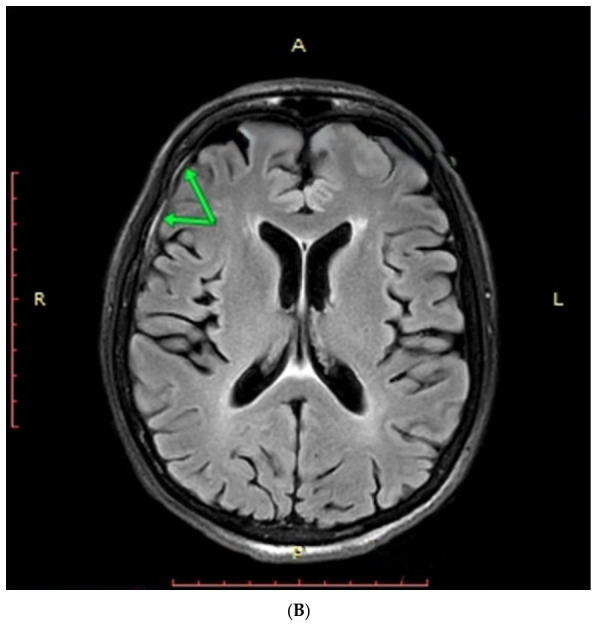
(A) Axial section of the brain on non-contrast computed tomography (radiological image of a right-sided subdural hematoma causing marked compression of the brain tissue—measurement of the hematoma width). (B) Axial section of the brain in T2 FLAIR sequence on 3T MRI (radiological image, follow-up after embolization with regression of the right-sided subdural hematoma—the hematoma bed is indicated by arrows). Own material. A—Anterior; L—Left; P—Posterior; R—Right.
